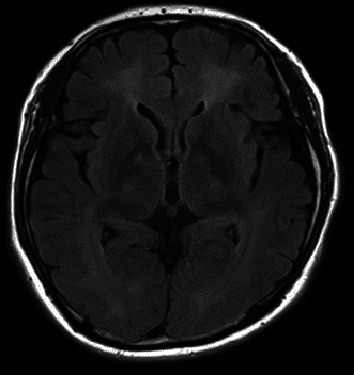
Magnetic resonance imaging (MRI) 28 days after the suicide attempt of case 1.
